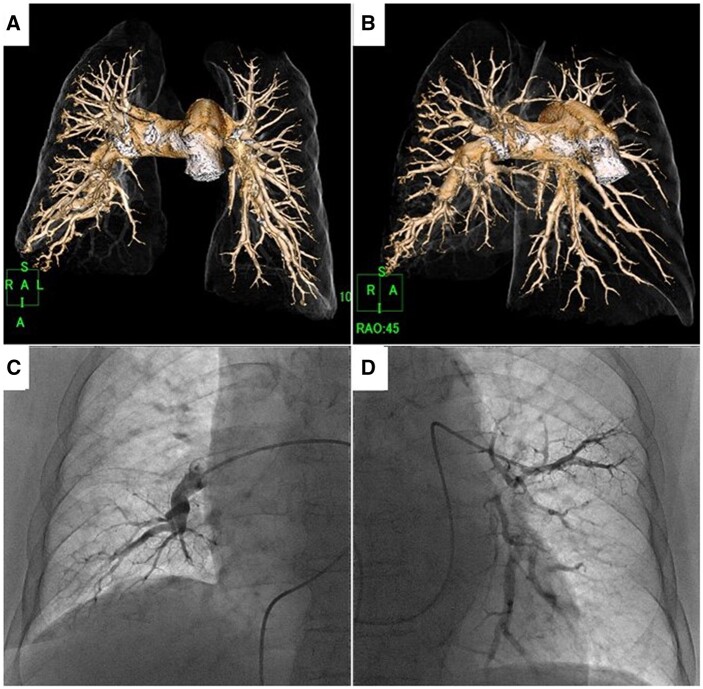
Contrast-enhanced computed tomography of the chest with three-dimensional reconstruction detected no pulmonary embolism (*A*; frontal view, *B*; right anterior oblique view). Pulmonary angiography showed no narrowing or obstruction of the pulmonary artery (*C*; right middle and lower lobe segment in frontal view, *D*; left lingual and lower lobe segment in frontal view).
